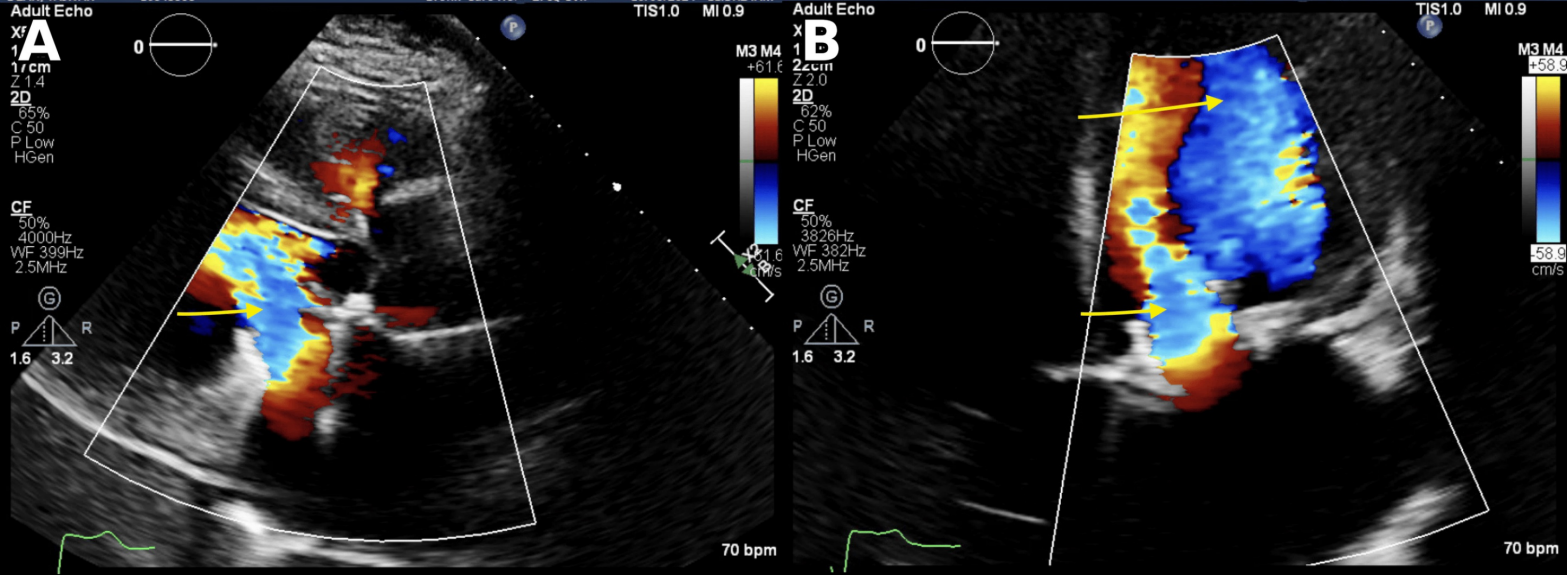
Transesophageal echocardiogram showing rergurgitant jet at mitral surface in parasternal long axis view (A) and posteriorly directed eccentric jet shown in apical view (B) Findings labelled by yellow arrows
